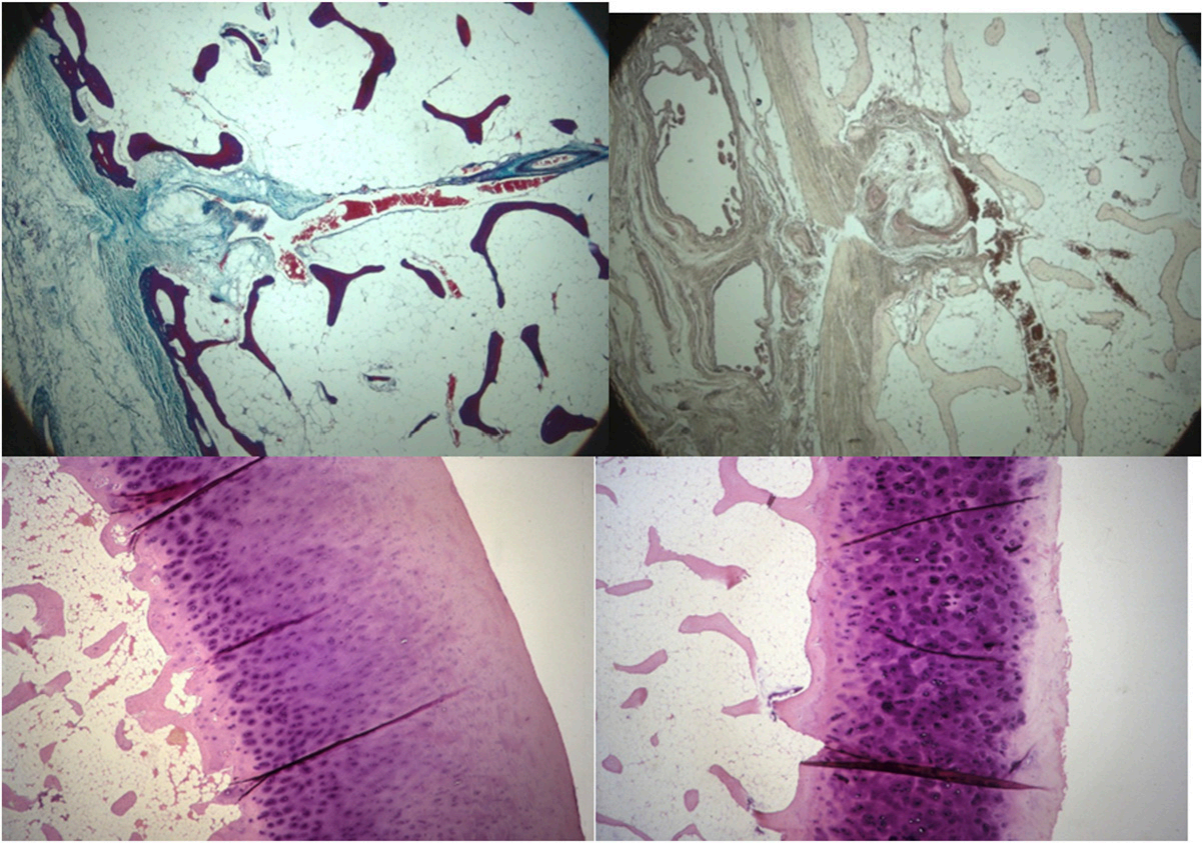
Upper pair: Cortex on the left, example of vessels seen on MRI in the subchondral plane about 1 cm below the tibial joint surface. Vascular distortion or possible choke valve as they reach the cortex. Goldner's trichrome x 20 and Masson's trichrome x 20. Lower pair: Left normal femoral head with plentiful subchondral vessels, right early osteoarthritic femoral head with none. H+E x 20.
